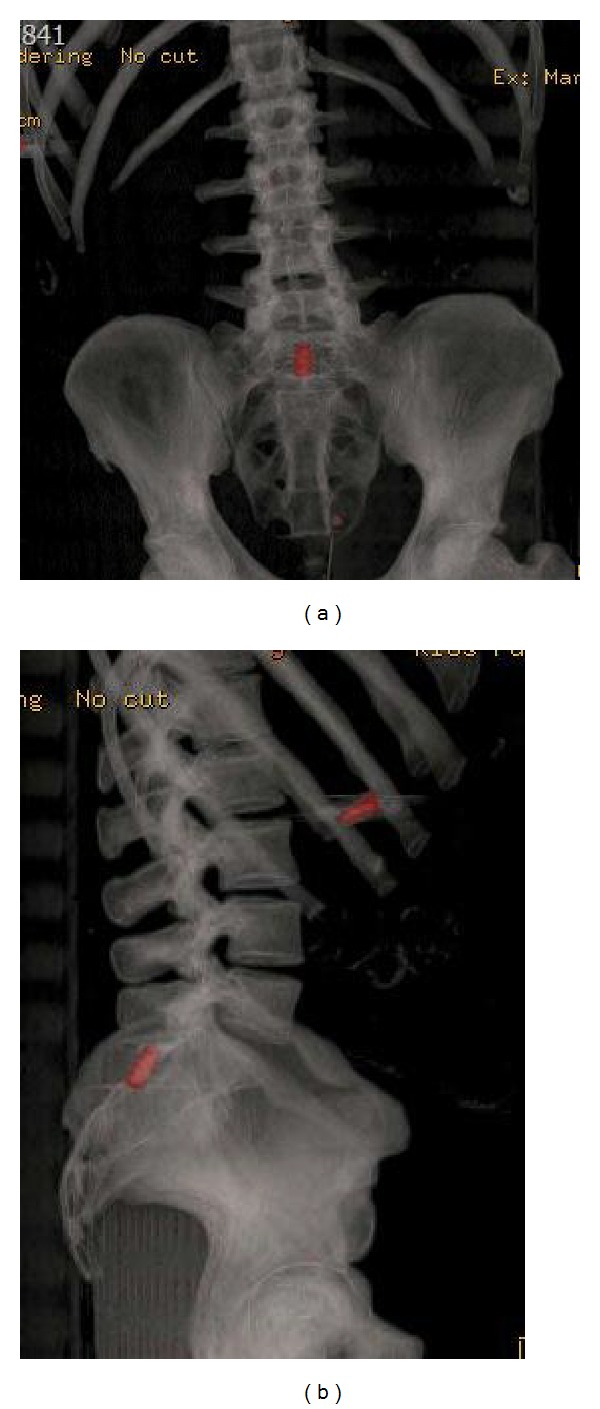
3D CT scan reconstruction.
